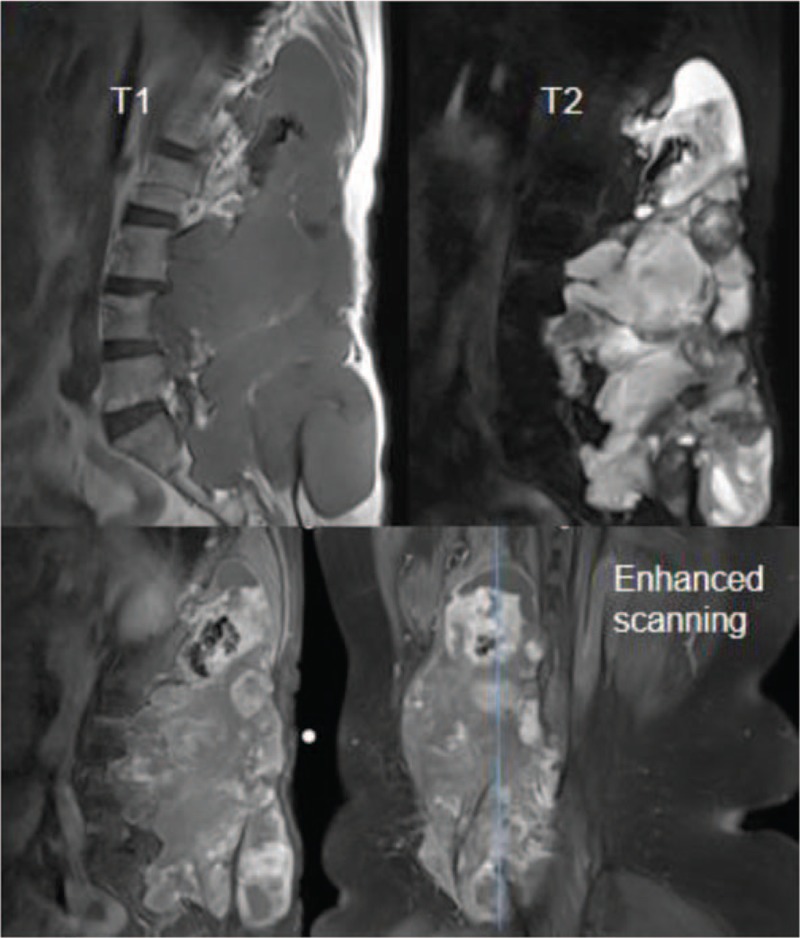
Magnetic resonance imaging showed a huge irregular mass shadow, with an unclear boundary of approximately 11 × 11 × 22 cm in size. There were uneven signals: low T1 signals were mixed with high T2 signals; multiple divisions were seen inside the mass; and multiple patchy high T1 and low T2 signals were present above the lesions. An enhanced scan indicated uneven reinforcement and obvious enhancement of the divisions in the lesions.
